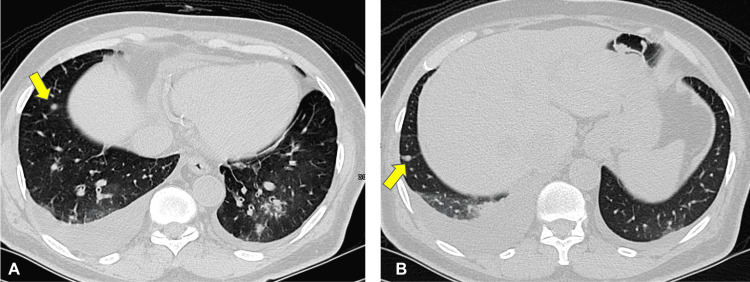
Baseline computed tomography images (A, B) just before starting treatment in late January 2019 shows multiple bilateral solid pulmonary nodules, largest measures 0.7 cm, highly suspicious for metastatic disease with bilateral pleural effusion.
